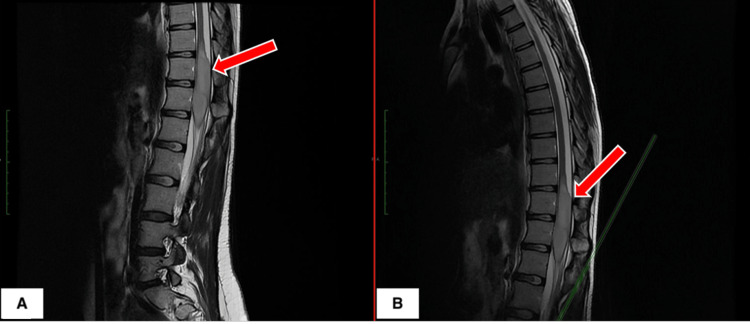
Preoperative MRI T2 sequence, (A) thoracic and (B) lumbar spinal cord, sagittal section showing T10-L1 intramedullary lesion with infiltrative character
